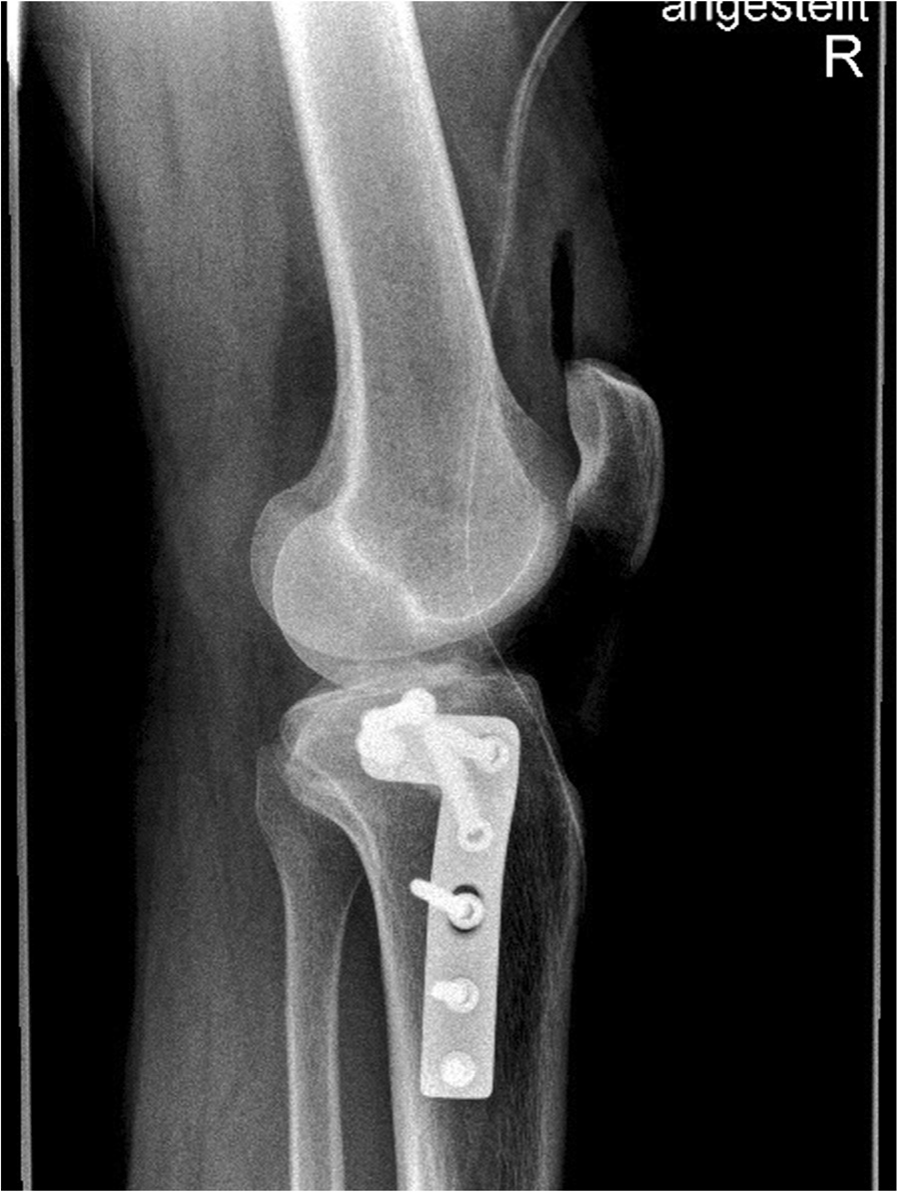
Postoperative lateral X-ray of the knee showing the reconstruction result.
